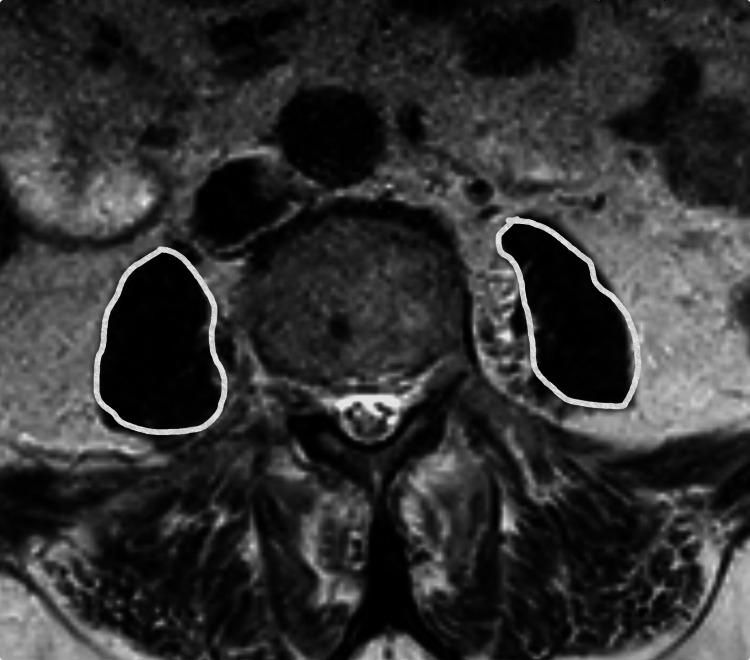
MR image used in measurements. The area marked in white shows the psoas muscle.
